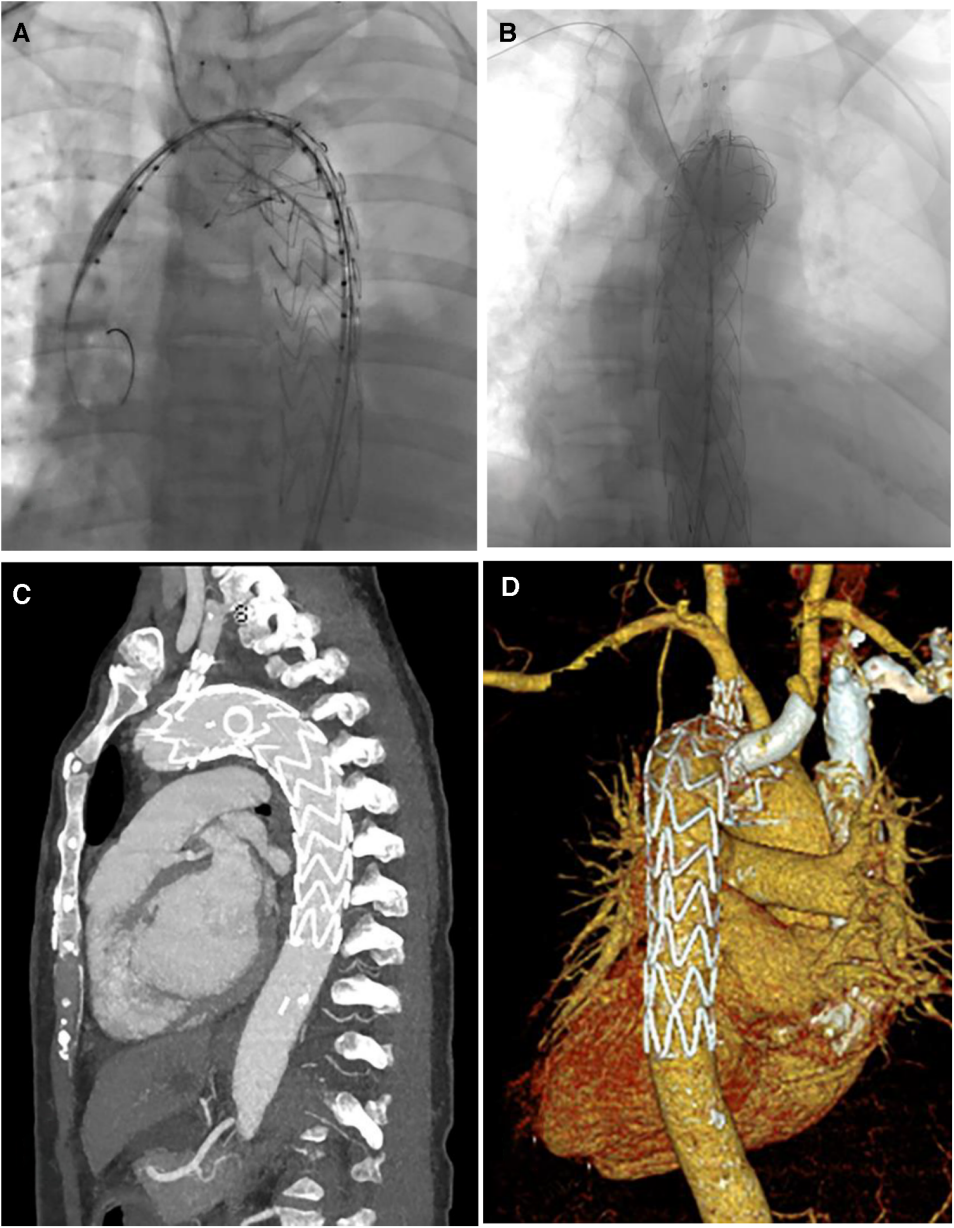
(A,B) Digital subtraction angiography in TEVAR of patient 4; (C,D) CT image remodeling 1 month after TEVAR.
